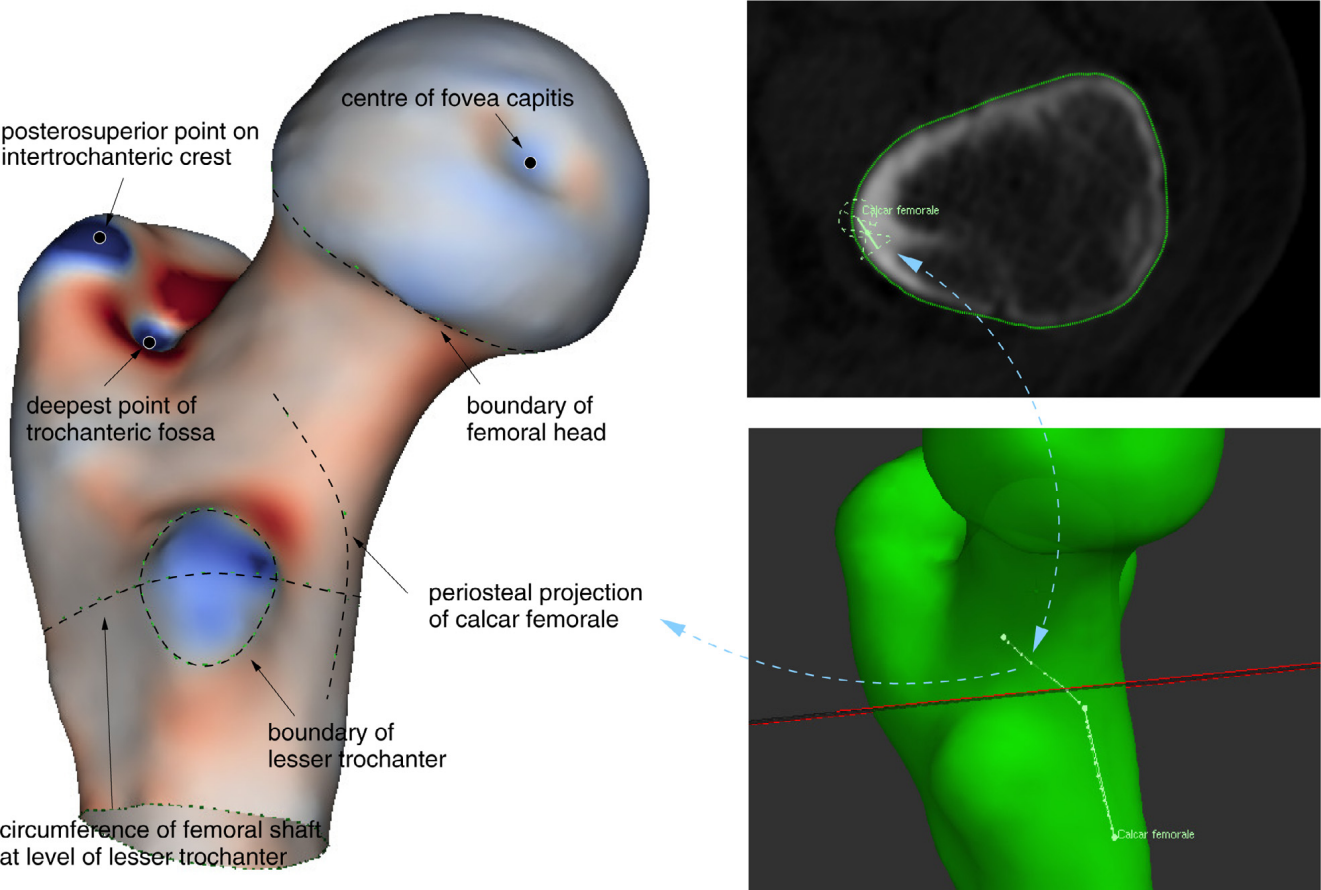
Point and curve landmarks used in the TPS-LM registration algorithm. Most of these landmarks are placed manually or semi-automatically with reference to the Gaussian curvature of the mesh. In the rendering on the left, Gaussian curvature is shown on a red–blue scale, with blue representing positive values and red representing negative values. The calcar femorale are traced manually on the green mesh (bottom right), the resulting curve is projected back into the original CT data (top right), and the curve is then edited on the green mesh until the projections align well with the calcar in the CT data. (For interpretation of the references to colour in this figure legend, the reader is referred to the web version of this article.)
